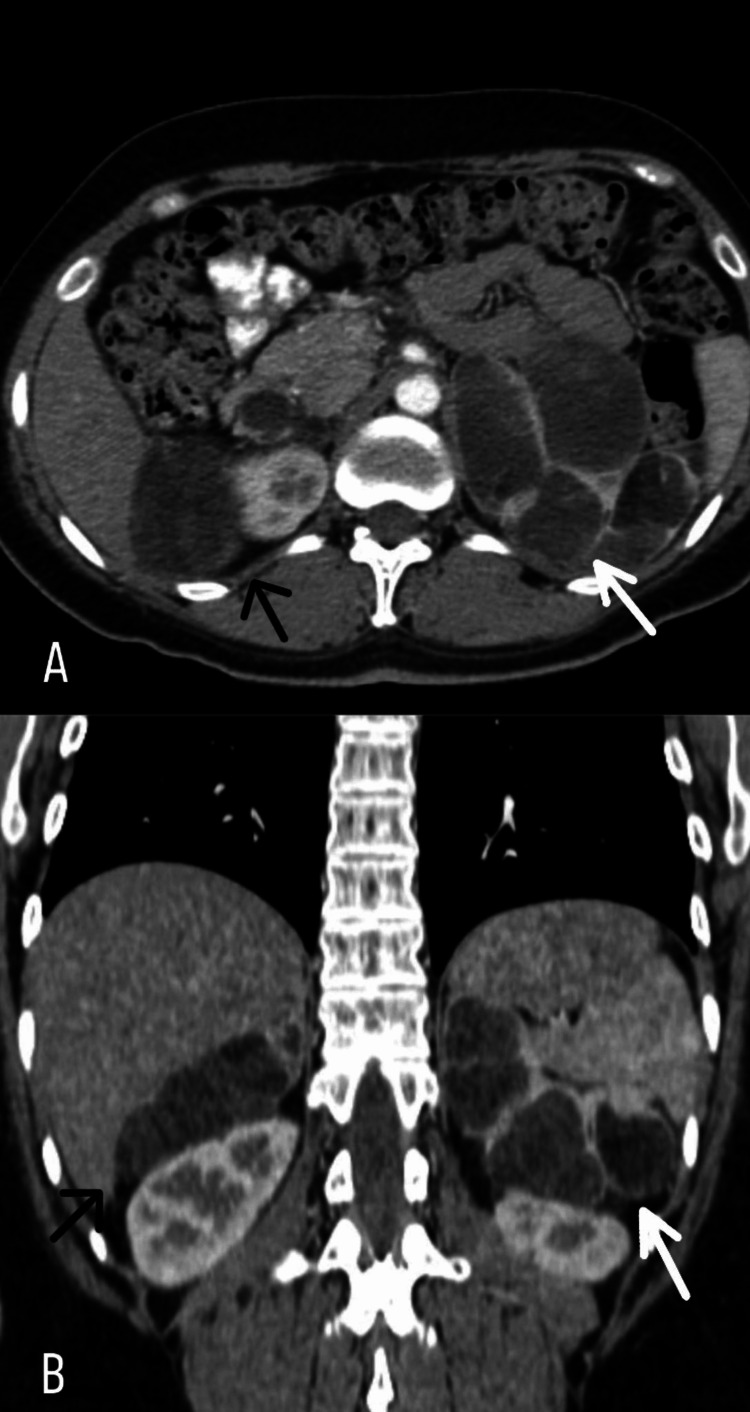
A: axial view, B: coronal view. Computed tomography (CT) scan uncovered bilateral adrenal masses characterized by a heterogeneous and multicystic nature. The right mass measured 8x5cm (black arrow) and the left mass measured 10x7.5cm (white arrow).
